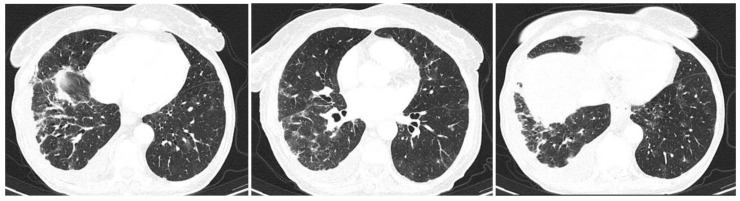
Chest CT scans one month prior to admission showing bilateral ground glass opacities of the lower lobes.
